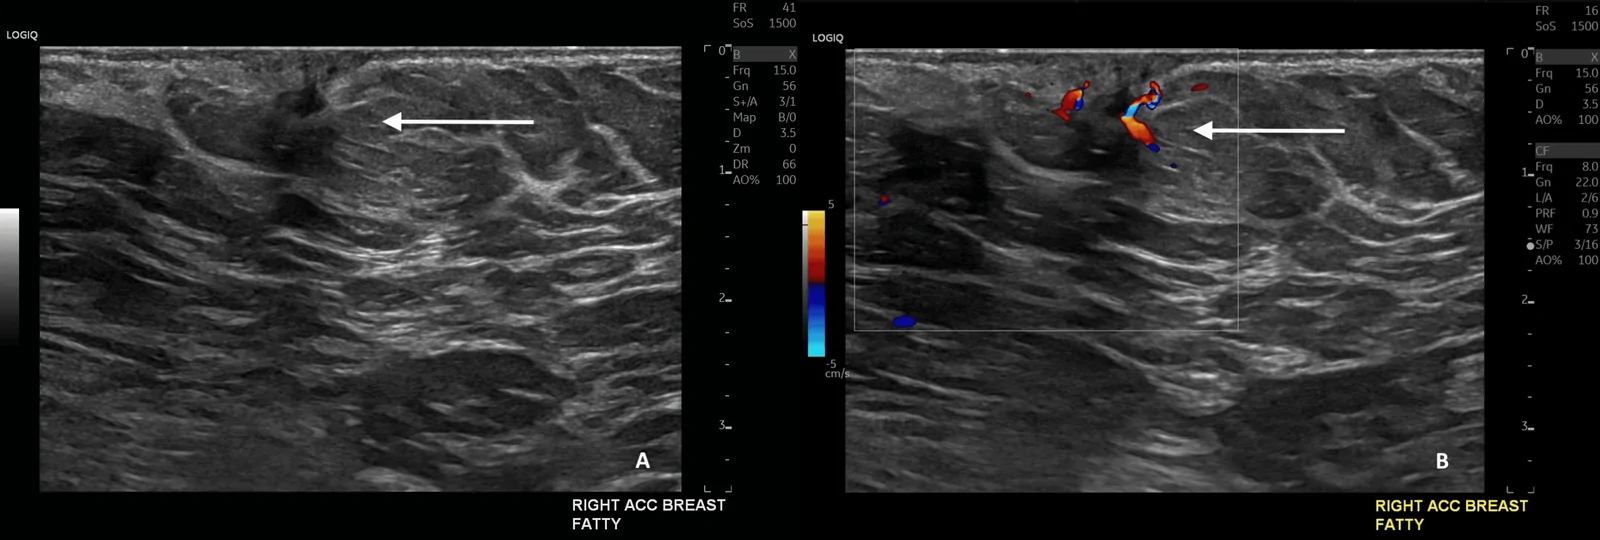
Ultrasound shows a 7mm hypoechoic, irregular spiculated lesion with evidence of skin involvement (A). Colour doppler demonstrates hypervascularity of the lesion (B).
